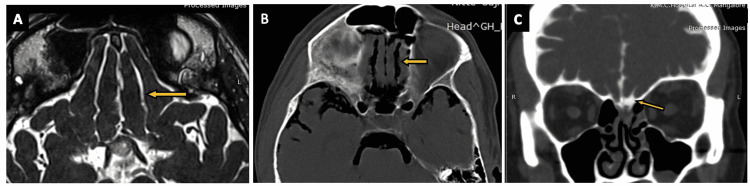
The olfactory cistern. (A) The olfactory cistern (arrow) as visualized on an axial section of the routine three-dimensional (3D) constructive interference in steady state (CISS) MRI sequence. (B) The olfactory cistern (arrow) as visualized on an axial section of CT brain (negative contrast) in a case of pneumocephalus. (C) The olfactory cistern (arrow) as visualized on a coronal section of CT cisternography in a case of suspected CSF rhinorrhea.
